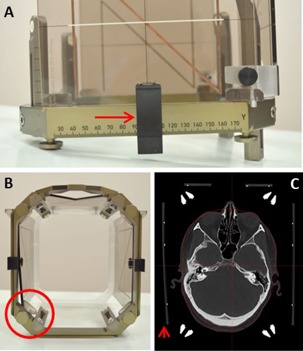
Fiducial indicator box accidentally misplaced at post‐GKR CT scan. Lateral view (a) of the stereotactic frame with the CT fiducial indicator box in an apparently normal position with the lateral clip adequately secured (red arrow). An inferior view (b) of the frame shows the right posterior locating pin of the indicator box displaced towards the midline (red circle) that resulted in an erroneous position of the fiducials in the CT scan ((c), arrowhead).
